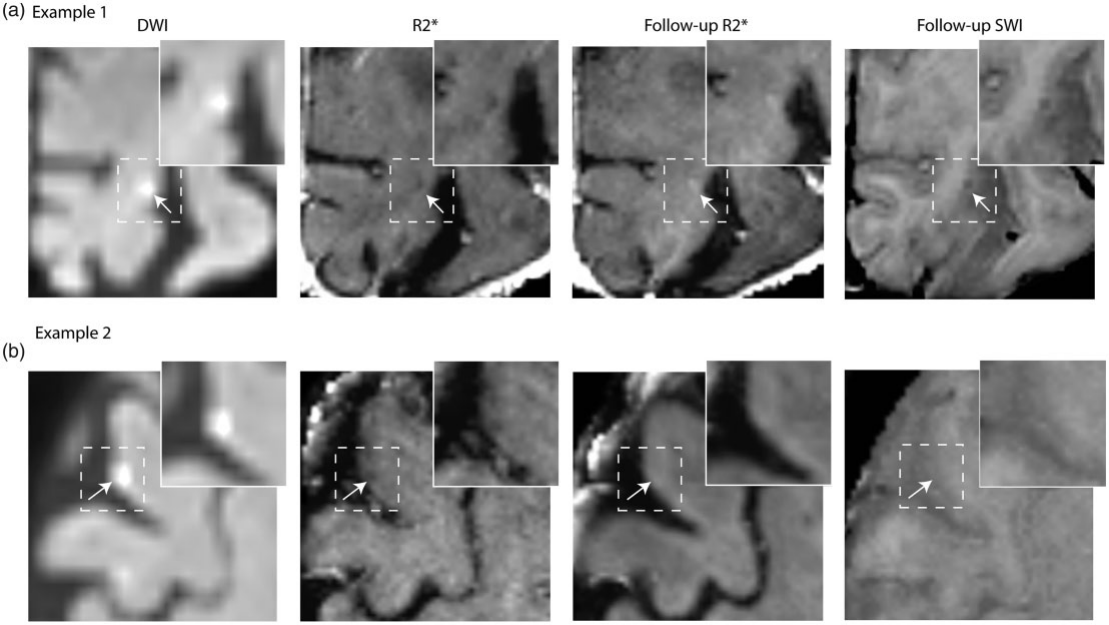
Examples of R2* signal change at the location of acute cortical microinfarcts. Acute cortical microinfarcts detected on diffusion-weighted imaging (DWI) trace images (shown here b = 3000), accompanied by a hypointense signal on R2* in the acute stage in both panel A and in panel B. On the last available follow-up MRI, the microinfarct in panel A is characterized by an increased signal on R2* and a decreased signal on SWI, indicative of iron accumulation, whereas in panel B no visible signal alterations were observed on either R2* or SWI images at the location of the microinfarct (B).
